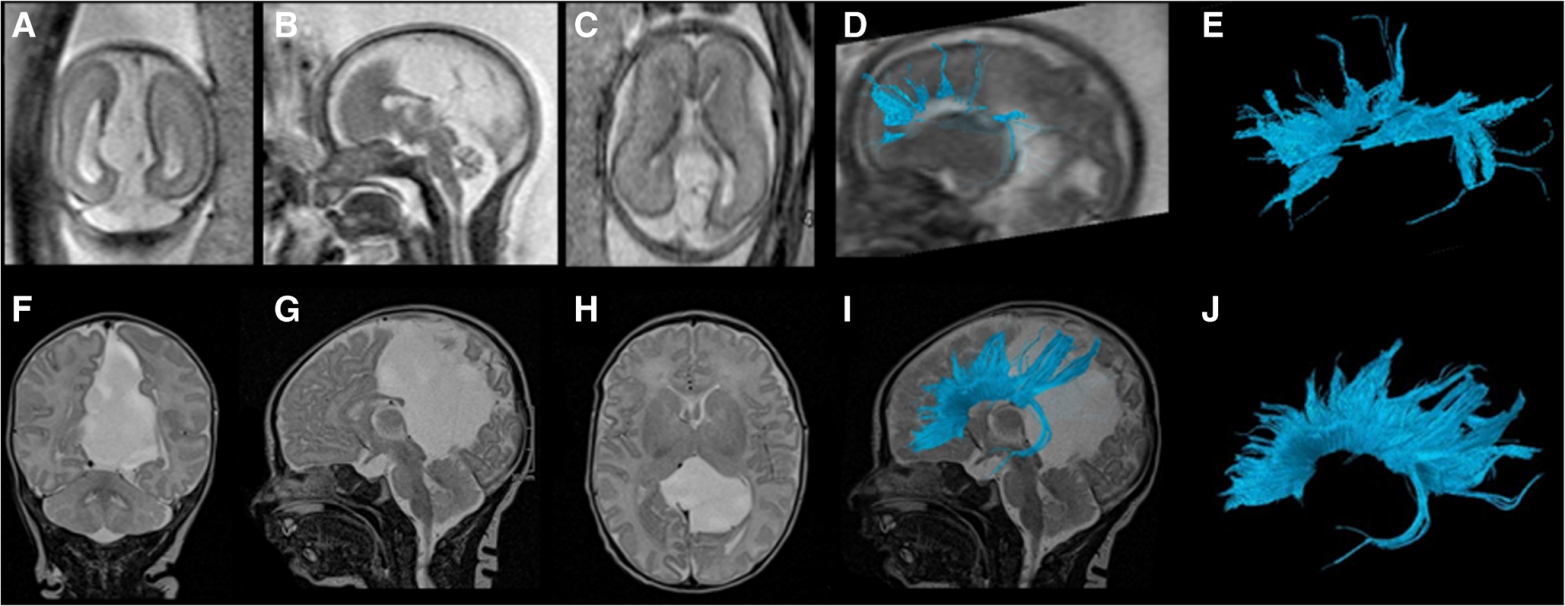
Fetal and postnatal corpus callosum segmentation in a boy (case 9) with a midline parieto-occipital cyst. a–e Gestational weeks 29+4. a Coronal T2-weighted (TR/TE 10,658/140 ms, flip angle 90°) MR image of the brain. b, c Sagittal (b) and axial (c) T2-weighted MR images of the brain with a midline posterior parieto-occipital cyst. d Overlay of the fiber tracts of the corpus callosum on a sagittal T2-weighted image. e The rostrum, genu, body and splenium are identifiable on this sagittal diffusion tensor image (DTI) of the corpus callosum in the fetal brain. f–j Age 5 days. f Coronal T2-weighted (TR/TE 3,070/134 ms, flip angle 180°) MR image of the brain. g Sagittal T2-weighted image. h Axial T2-weighted image of the brain with a midline posterior parieto-occipital cyst. i Segmented sagittal tractography of the corpus callosum. j The rostrum, genu, body and splenium are identifiable on sagittal DTI of the corpus callosum in the 5-day-old postnatal brain. *TE* echo time, *TR* repetition time
